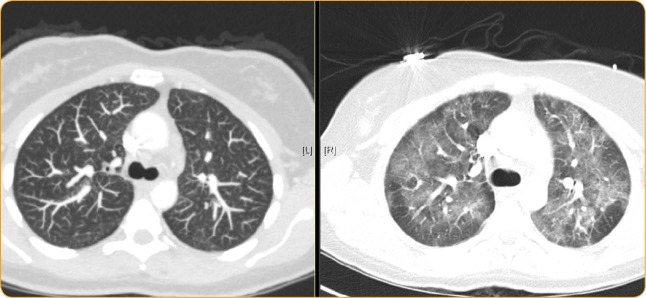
The CT scan on the left was taken prior to starting treatment with crizotinib. The CT scan on the right is an example of pneumonitis occurring 3 weeks after starting crizotinib.
